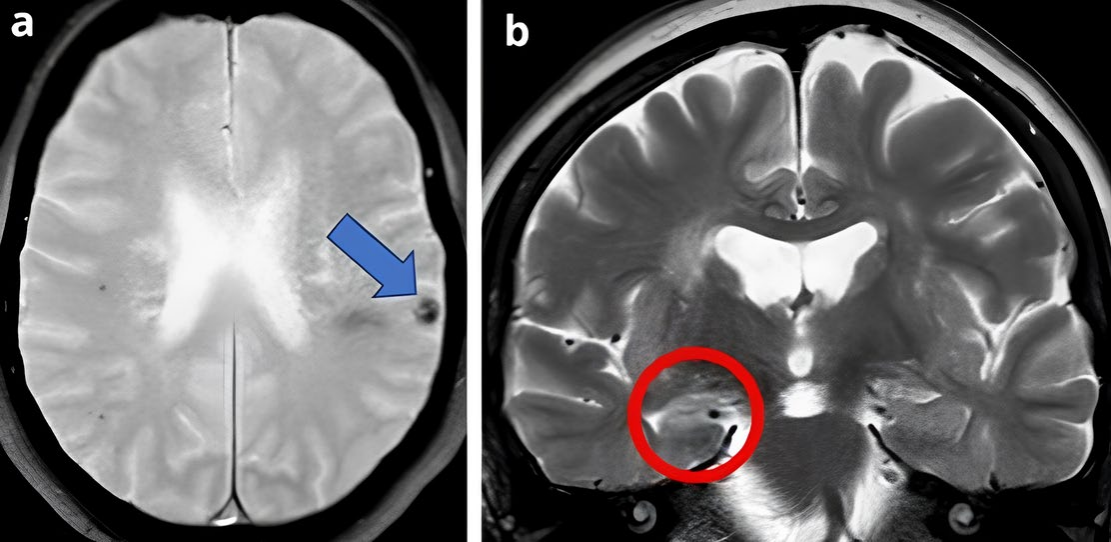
Magnetic resonance images in a patient with MTLE‐HS plus NCC. (A) Axial, T2‐gradient‐echo image showing a hypointense nodular lesion in the left parietal cortex (blue arrow), consistent with calcified neurocysticercosis. (B) Coronal, T2‐weighted image showing increased signal intensity and atrophy of the right hippocampus (red circle), compatible with hippocampal sclerosis.
